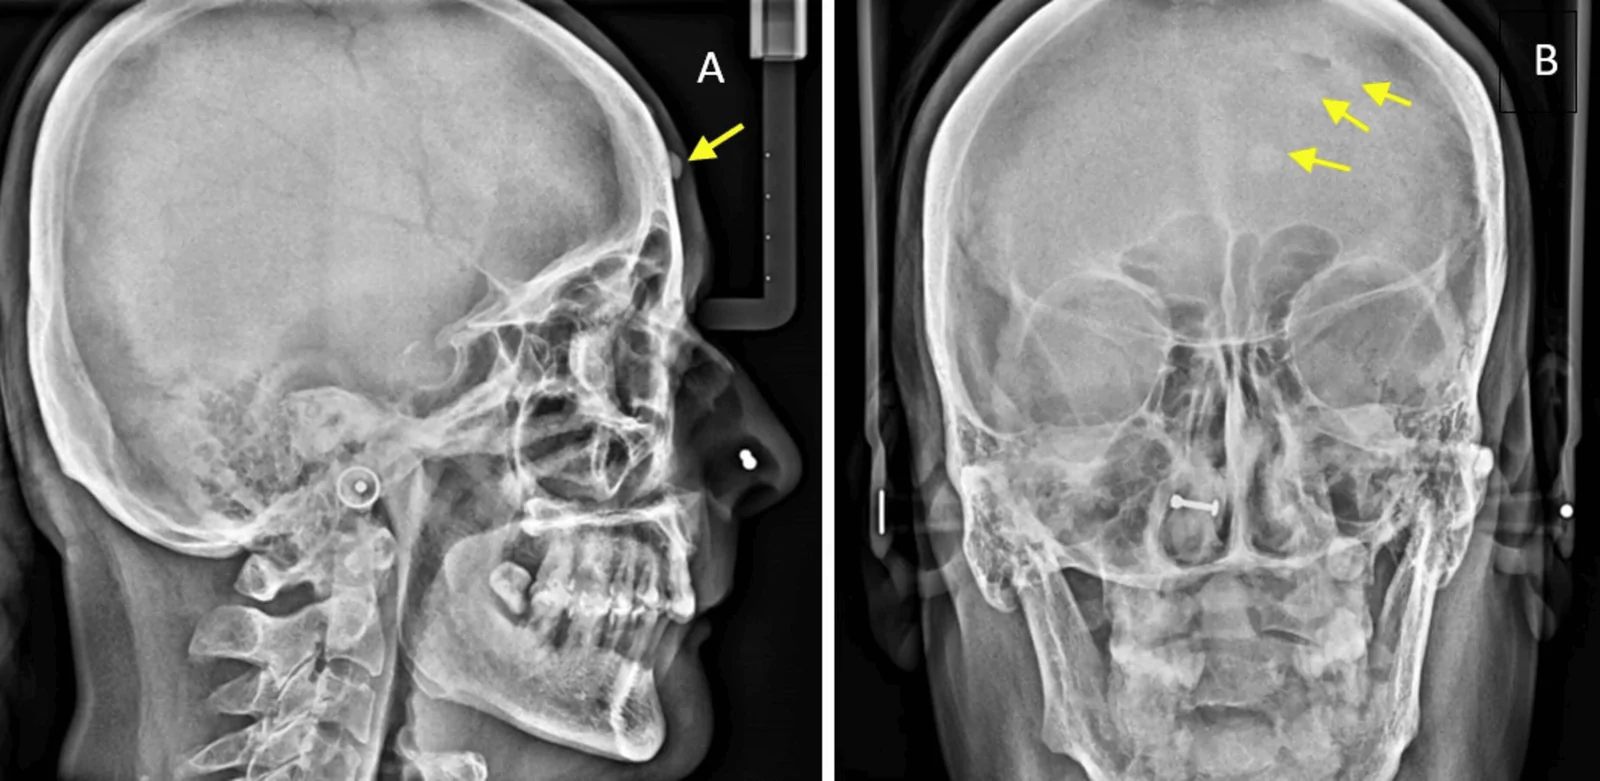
(A) Lateral cephalometric radiograph revealed a radiopaque mass attached to the outer cortical plate (yellow arrow). (B) Anteroposterior skull view revealed three discrete radiopacities on the frontal skull bone of varying diameters (yellow arrows) Patient informed consent was obtained for the publication of her radiographic images. The declaration of Helsinki is followed in this case report
